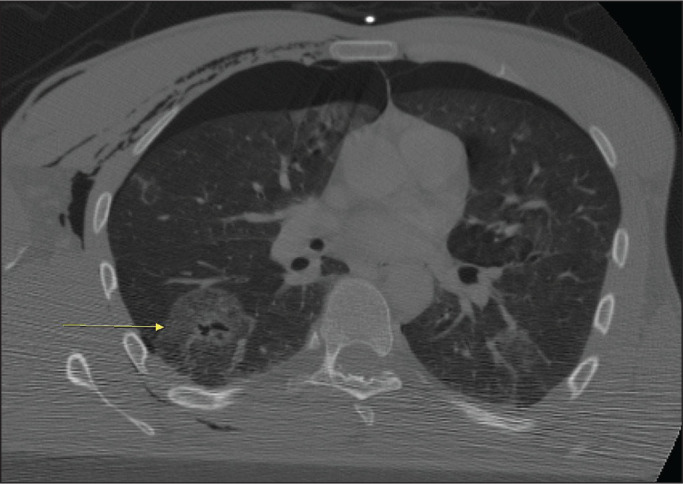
CT scan showing pulmonary contusion (arrow) in a patient with traumatic
